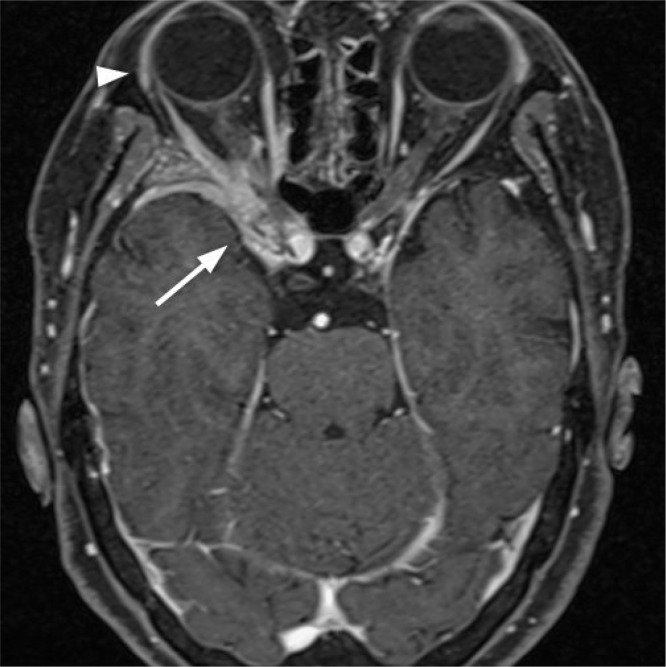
Fat-suppressed, postcontrast MR T1-weighted image shows an enhancing intraorbital mass (arrow) with no evidence of lacrimal involvement (arrowhead).
